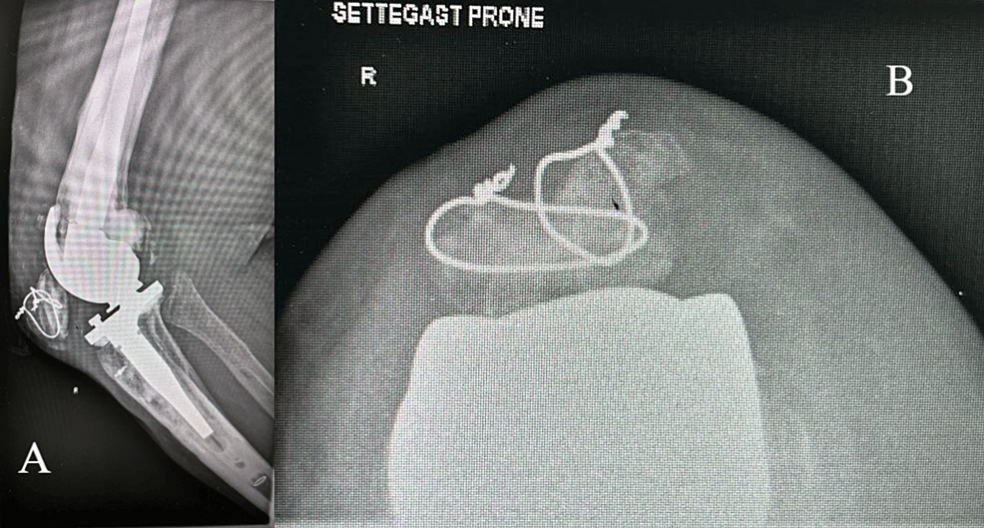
Preoperative X-rays of the knee demonstrating the periprosthetic patella fracture. (A) Lateral view of the knee. (B) Settegast prone view of the patella.
